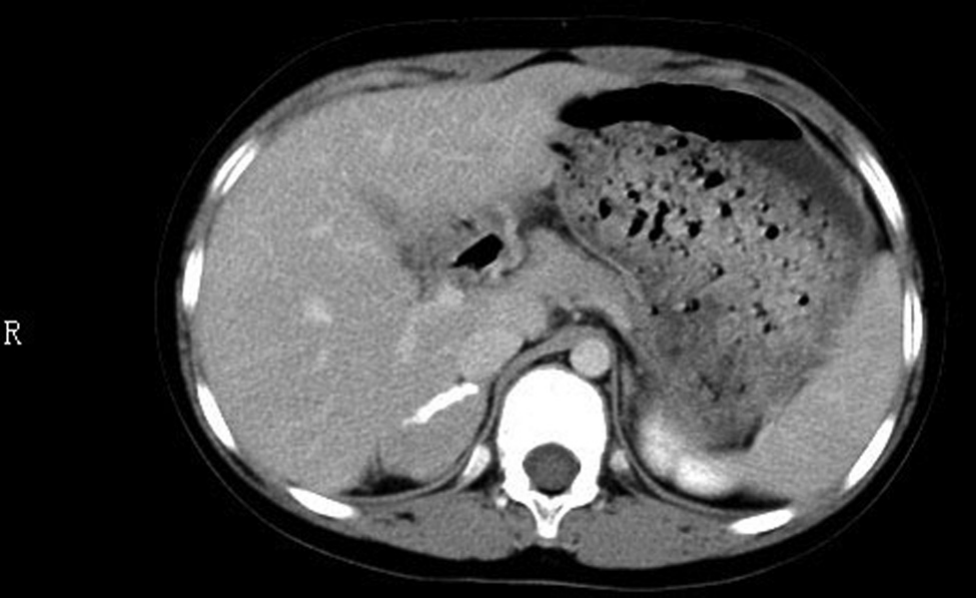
Enhanced CT scan of the patient’s abdomen showed a mass of 139.7 mm × 17.6 mm × 20.3 mm in the right adrenal gland.
